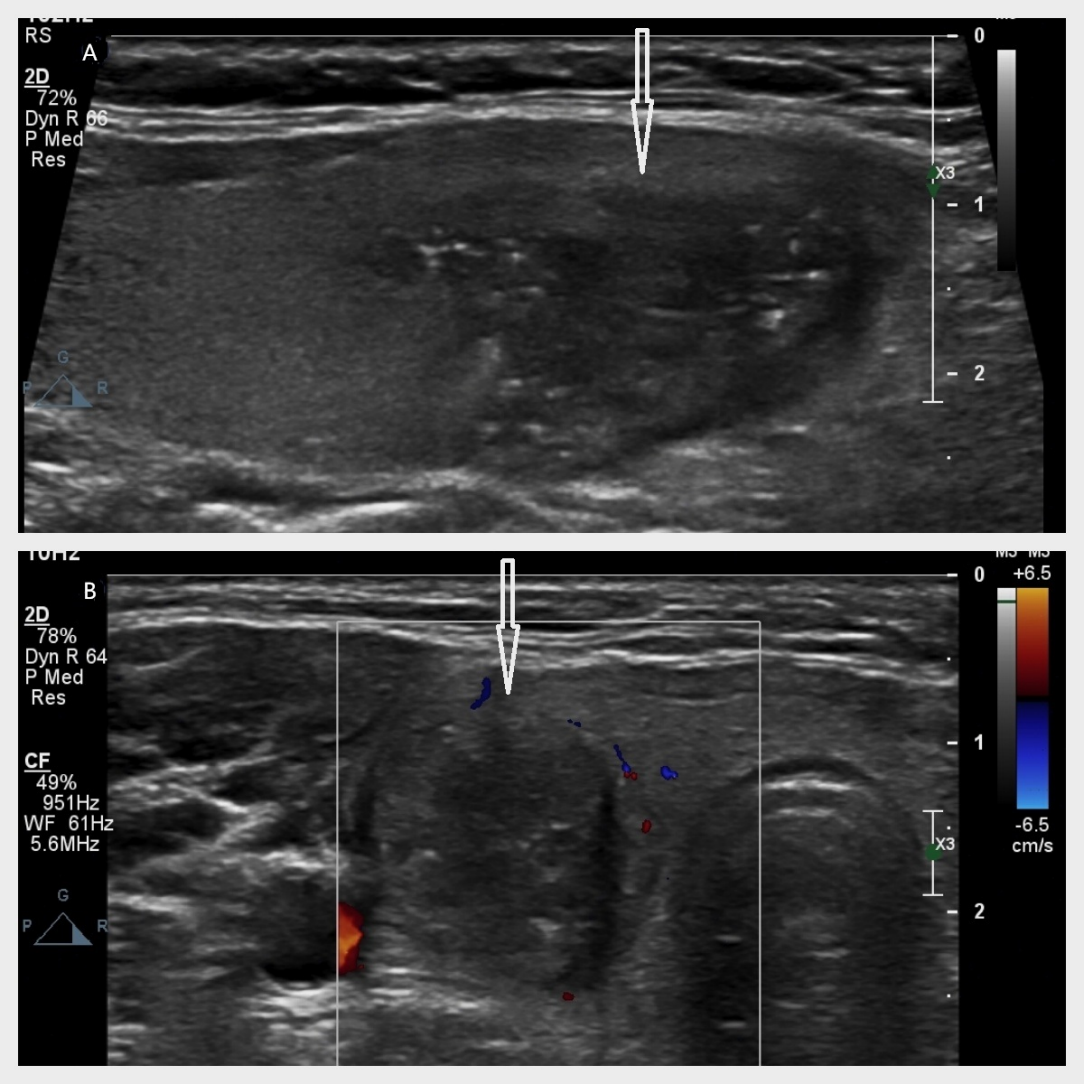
(A–B) A 30-year-old female with a pathologically confirmed thyroid nodule (papillary carcinoma). Ultrasound shows a hypoechoic nodule (arrows indicate) in the right thyroid lobe with the following features: irregular shape (no taller-than-wide), entirely solid composition, scattered punctate hyperechoic foci, partially discernible margins, and dimensions of 30.5 × 20.8 × 14.4 mm. No posterior acoustic features observed. Classification: classical C-TIRADS 4c, modified C-TIRADS 4c, K-TIRADS 5.
